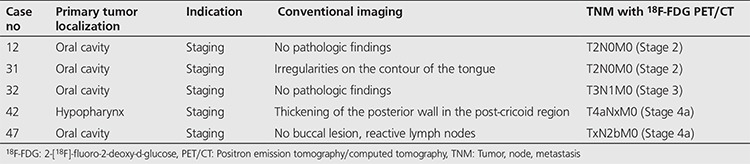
Tumor, node, metastasis staging with 18F-FDG positron emission tomography/computed tomography
